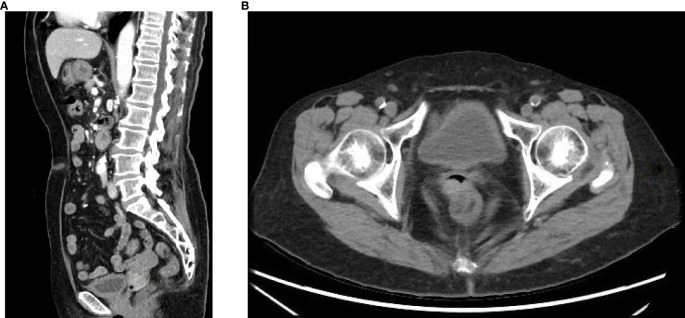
The pelvic CT scan showed that the cervical lesion had shrunk after neoadjuvant therapy.
